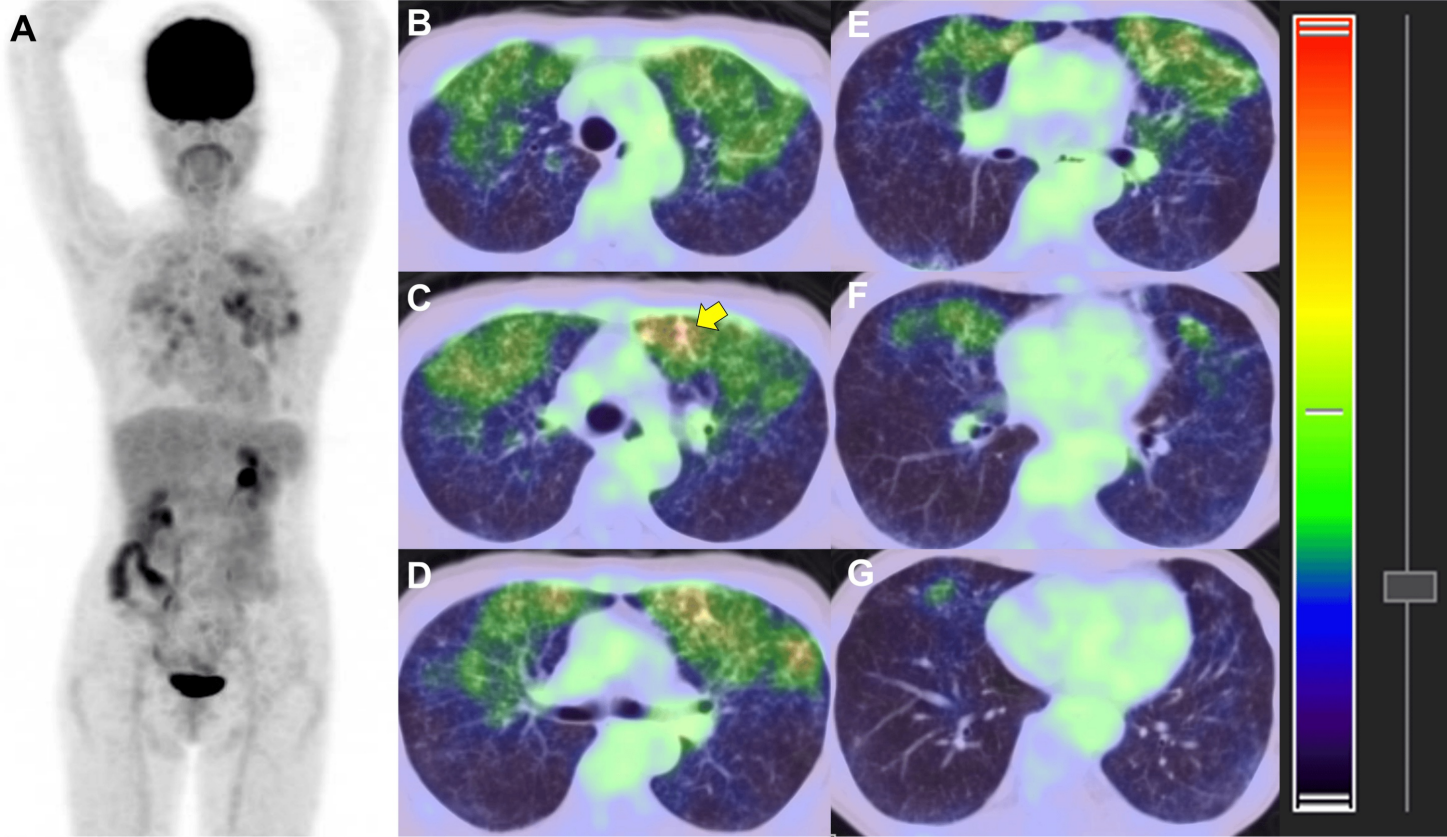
[¹⁸F]FDG-PET/CT (A) Maximum intensity projection (MIP) image demonstrates diffuse increased [¹⁸F]FDG uptake throughout both lungs. (B-G) Fused positron emission tomography/computed tomography (PET/CT) images show increased uptake corresponding to the distribution of the diffuse centrilobular nodules on CT. The most intense uptake is observed in areas of nodular confluence in the left lingula (yellow arrow), with a maximum standardized uptake value of 3.8. For both the MIP and axial images, the standardized uptake value display range was set from 0 to 4, and fused images were displayed with 70% CT and 30% PET weighting. [¹⁸F]FDG: 2-deoxy-2-[¹⁸F]fluoro-D-glucose
